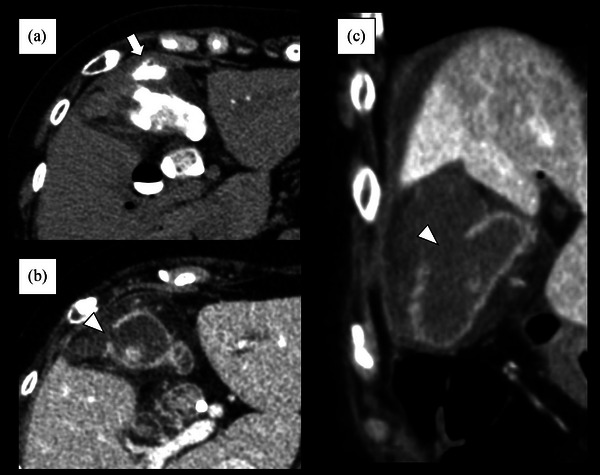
Findings of computed tomography performed after peroral cholangioscopy‐guided lithotripsy; (a) Plain CT images taken immediately after peroral cholangioscopy‐guided lithotripsy showing leakage of contrast agent into the subserous space of the gallbladder wall (white arrow) and marked edematous thickening of the gallbladder wall; (b,c) Contrast computed tomography images taken the day after peroral cholangioscopy‐guided lithotripsy showing a gallbladder wall defect (white arrowhead) in the gallbladder fundus with pericholecystic fluid.
